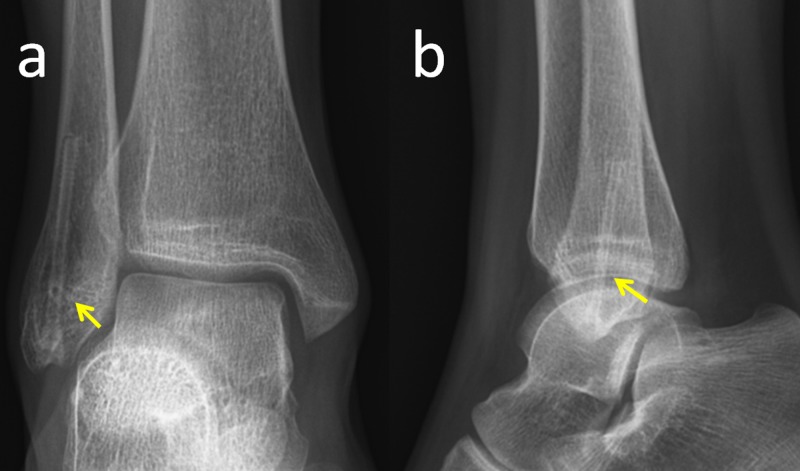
Postoperative radiographs Final ankle radiographs of the patient, two years after the operation. Yellow arrows show the shadow of the screw.
